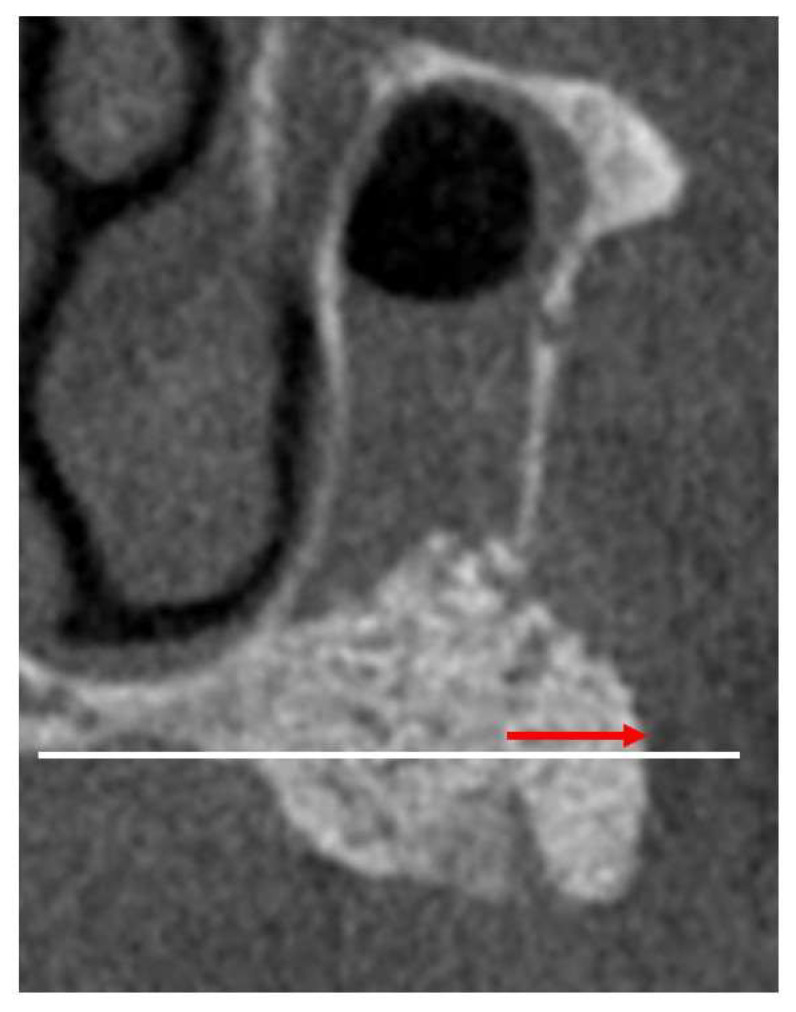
A CT scan showing the presence and amount of bone graft displacement (mm). An extended line connects the bilateral palatine bones (white line) in the coronal section at the lateral open window. The bone graft displacement was measured parallel to it (red arrow).
